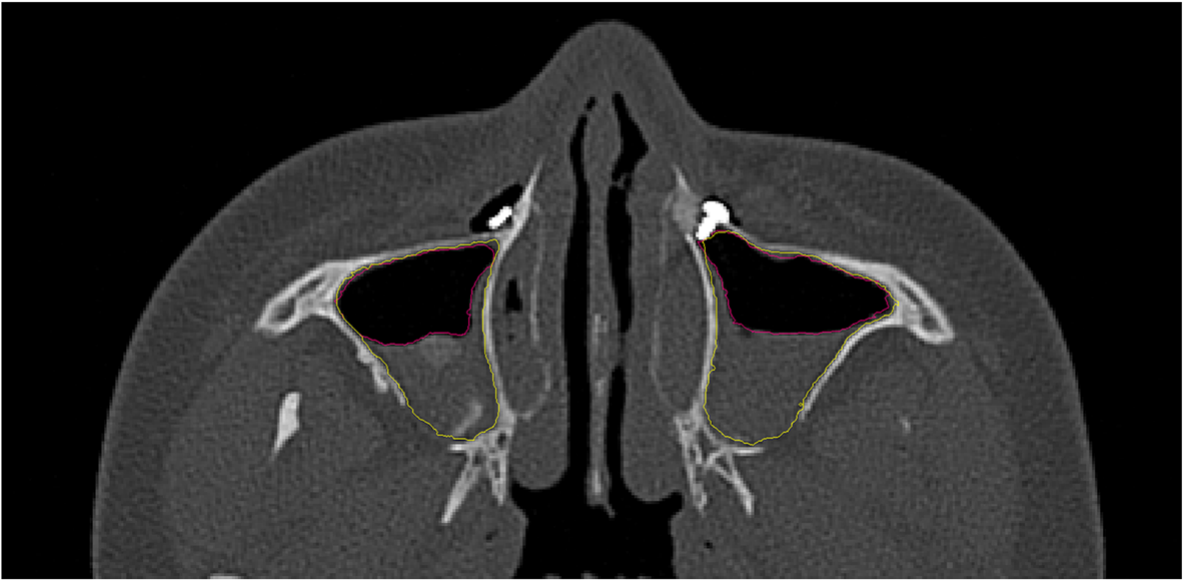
Borders of the empty spaces (red) within the maxillary sinus (yellow) according to the white gray values were identified on all CT scans at the level of the maxillary sinus
